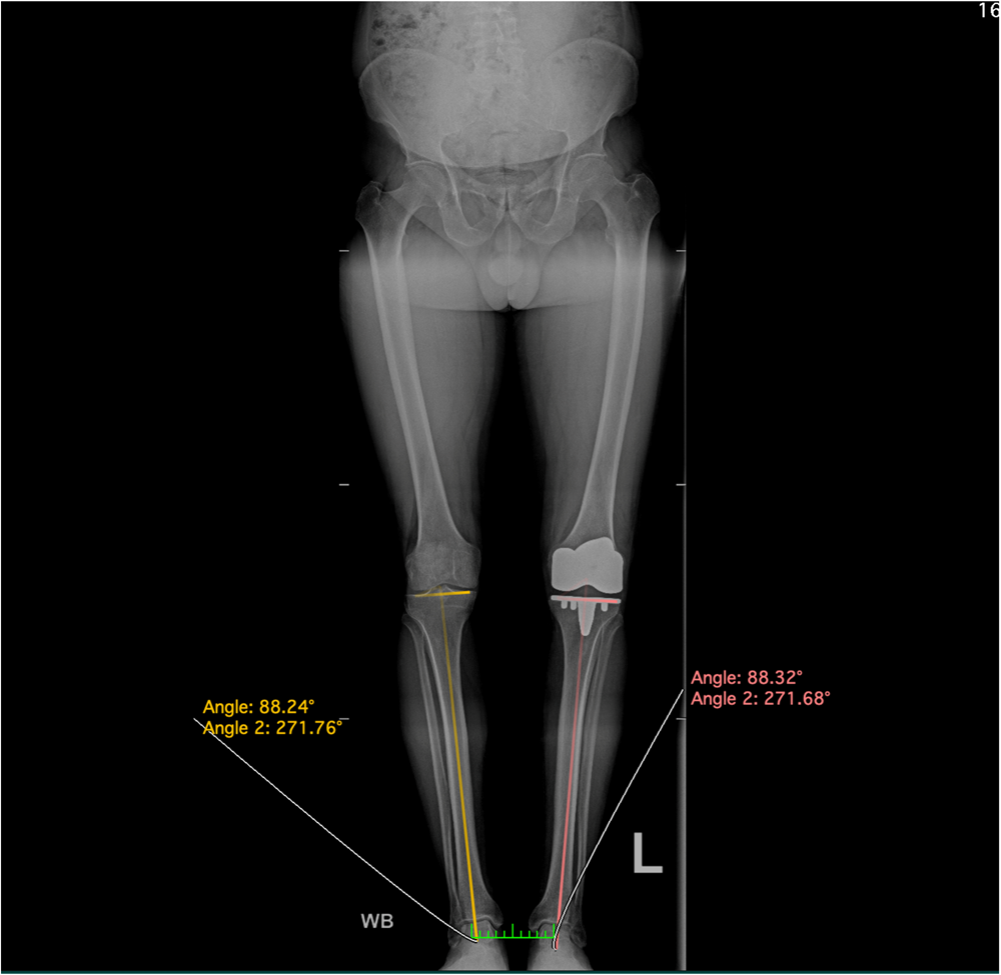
Postoperative radiograph demonstrating restoration of constitutional lower limb alignment, CPAK phenotype and medial proximal tibial angle.
